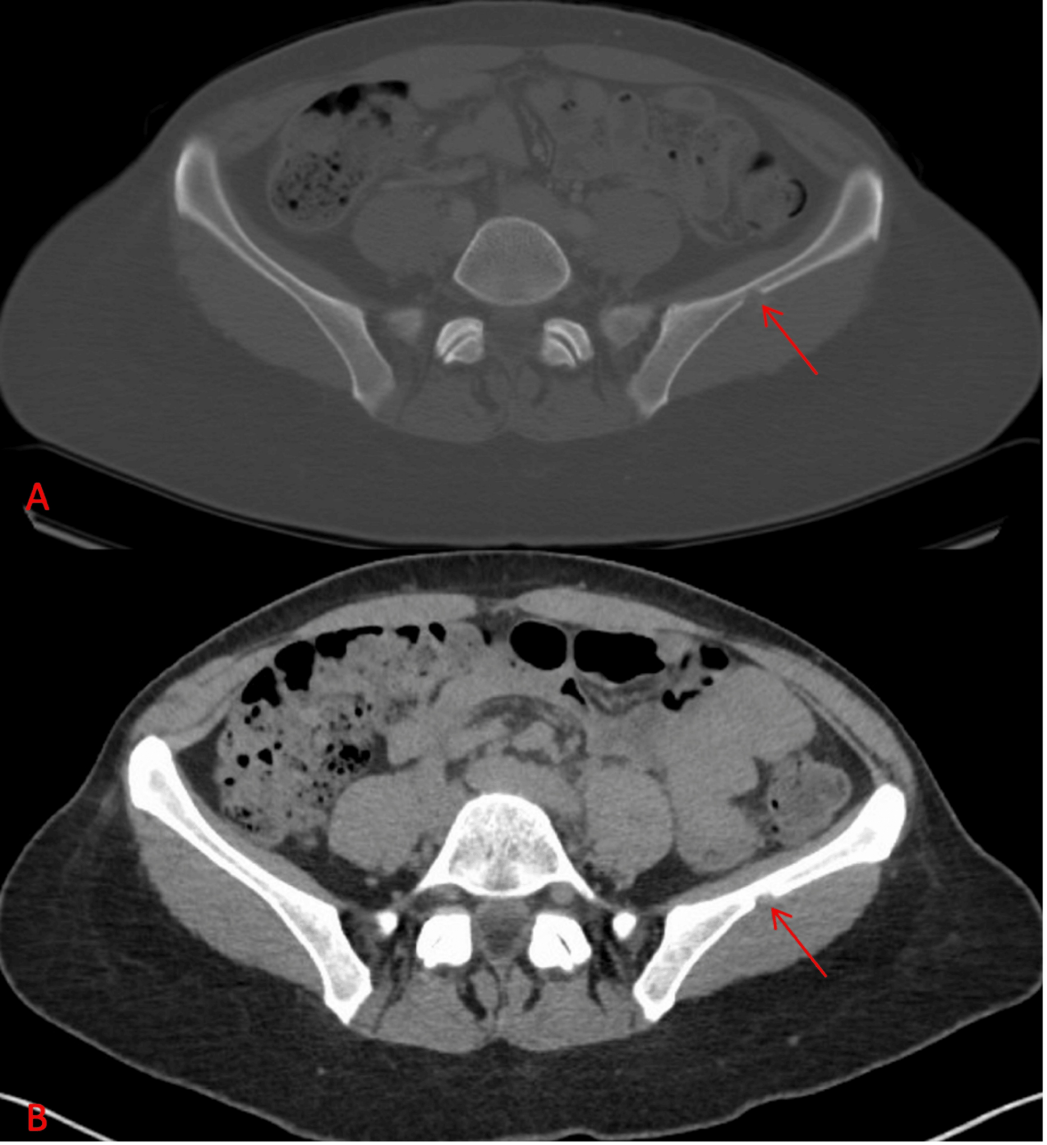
CT of the abdomen and pelvis without contrast (axial bone window (A) and axial abdominal window (B)) with an incidentally found lucent lesion in the left iliac bone (red arrows) CT: computed tomography
